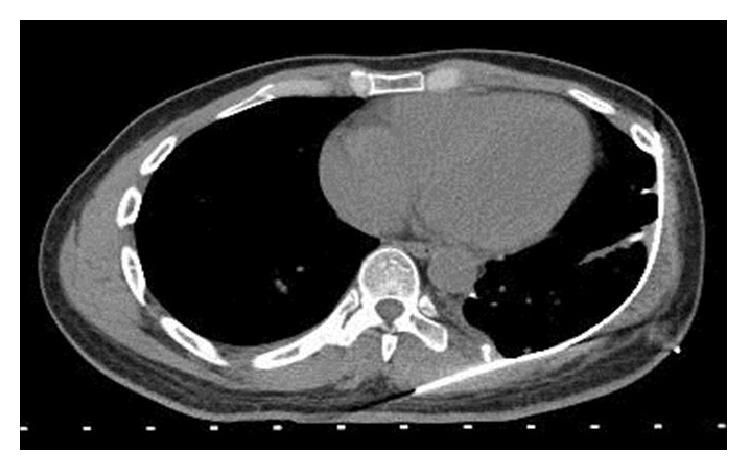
Follow-up axial CT chest without contrast demonstrating removal of pleural soft tissue mass, skeletal lesions, and titanium mesh chest wall reconstruction.
